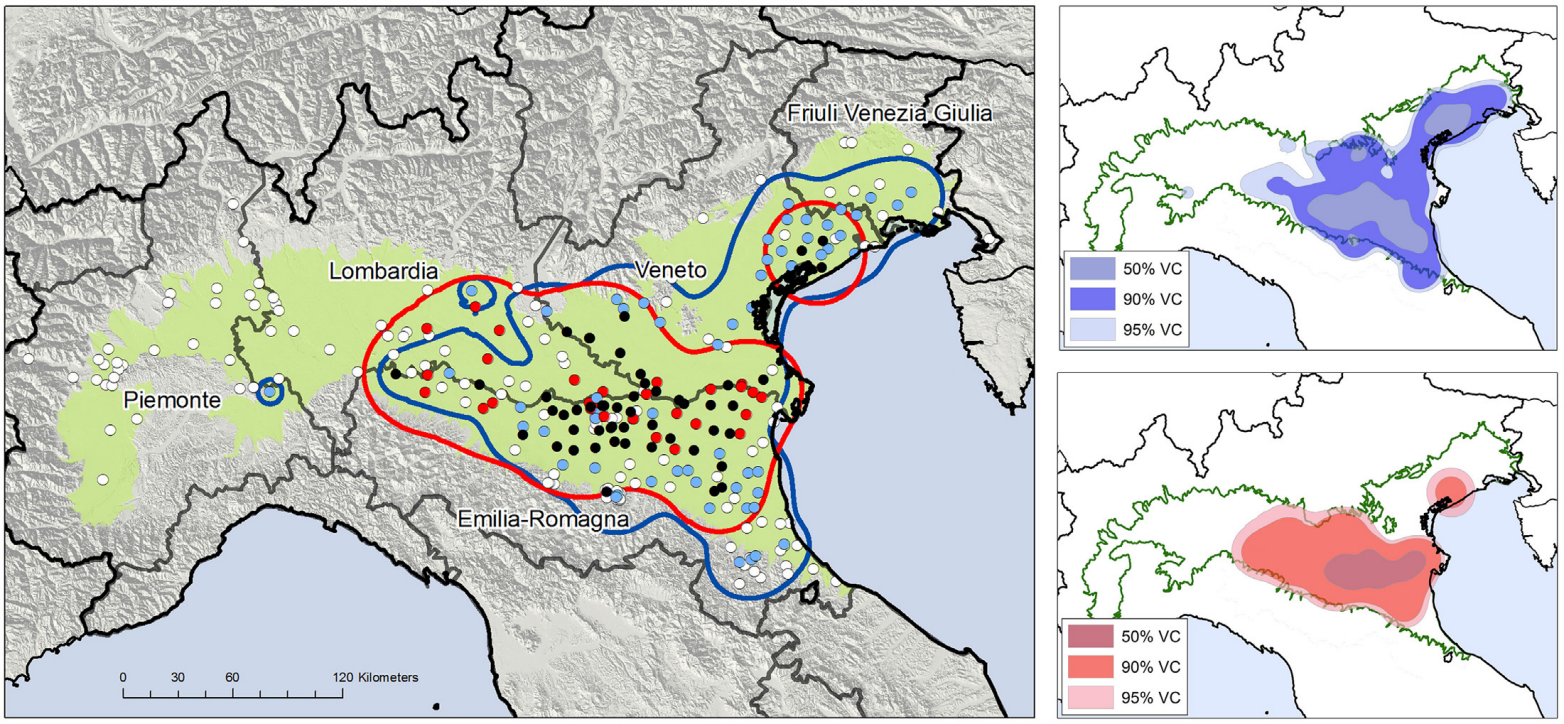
WNV and USUV detections. Surveyed area with sampling stations and locations of virus detection (red, WNV-positive; azure, USUV-positive; black, WNV- and USUV-positive) and borders of the WNV (red line) and USUV (azure line) circulation areas. On the right: volume contour (VC) of the WNV-circulation area (red) and of the USUV-circulation area (blue) estimated by kernel density estimation (KDE). The plain area and plain limit are reported in green.
